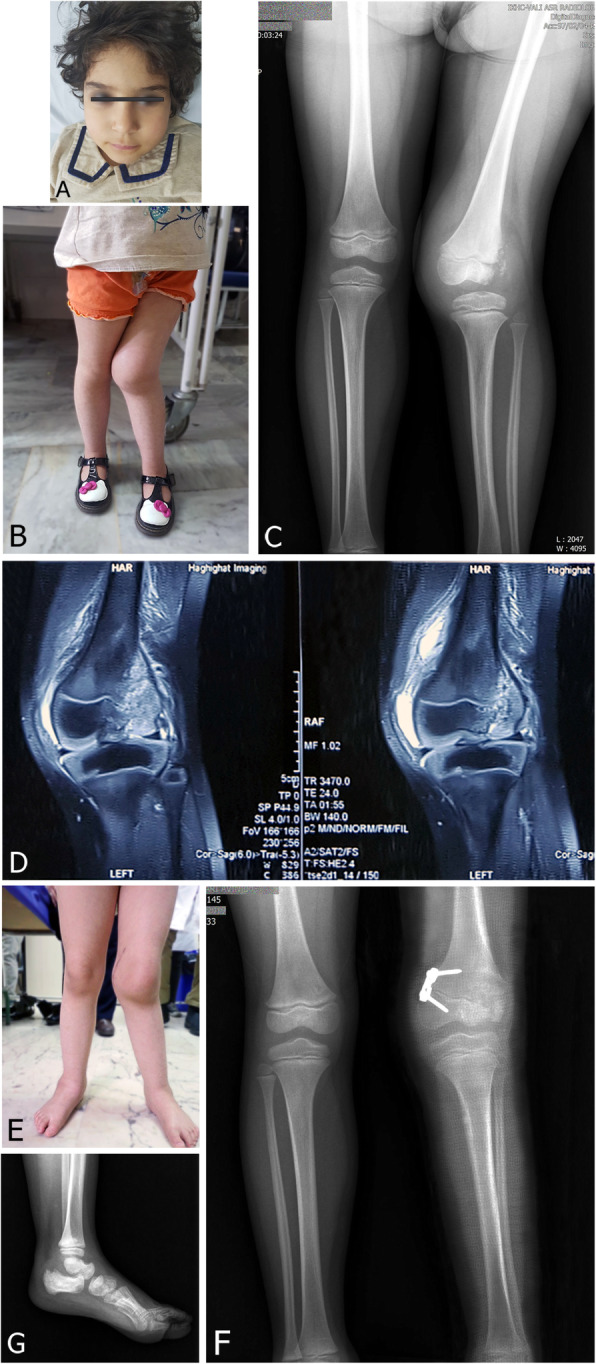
A five-year-old female (case 5) with HSAN type V. a No facial stigmata are present. b Clinical photograph at presentation, with massive knee effusion, left genu valgum, and difficulty walking. c Standing anteroposterior radiograph at presentation shows bone resorption in the lateral femoral condyle, increased joint space medially, and soft-tissue shadow indicative of knee effusion. d T2-weighted fat-saturated coronal magnetic resonance (MR) images show a lesion in the lateral femoral condyle, which suggests that the primary injury might have been a displaced Salter-Harris type IV lateral femoral condyle fracture. e Clinical photograph, 16 months postoperative. Left knee alignment has improved. Note that the incision is healed completely, and also the right ankle is swollen. f Standing AP radiograph at the same visit, showing an improved alignment of the left knee, consolidation of the initial lateral bone lucency, and improved joint congruency. The patient uses elastic bandages to decrease the swelling. g Lateral radiograph of the right ankle. Soft-tissue swelling and a sclerotic calcaneus are visualized, which indicate a probable bony injury. Short-term bracing was done for her ankle
